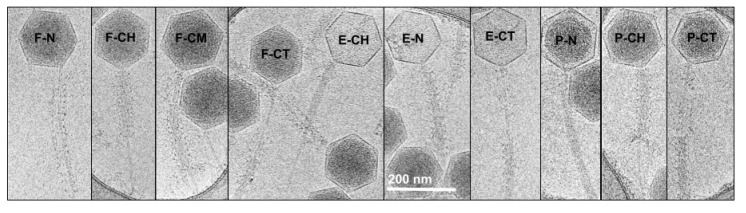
Representative members of cryo-EM-based categories of purified phage G. Particles are labeled as follows: F—DNA full; P—partial DNA; E—empty; N—non-contracted; CH—contracted near head; CM—contracted middle; CT—contracted at tip, as shown by the representative images.
